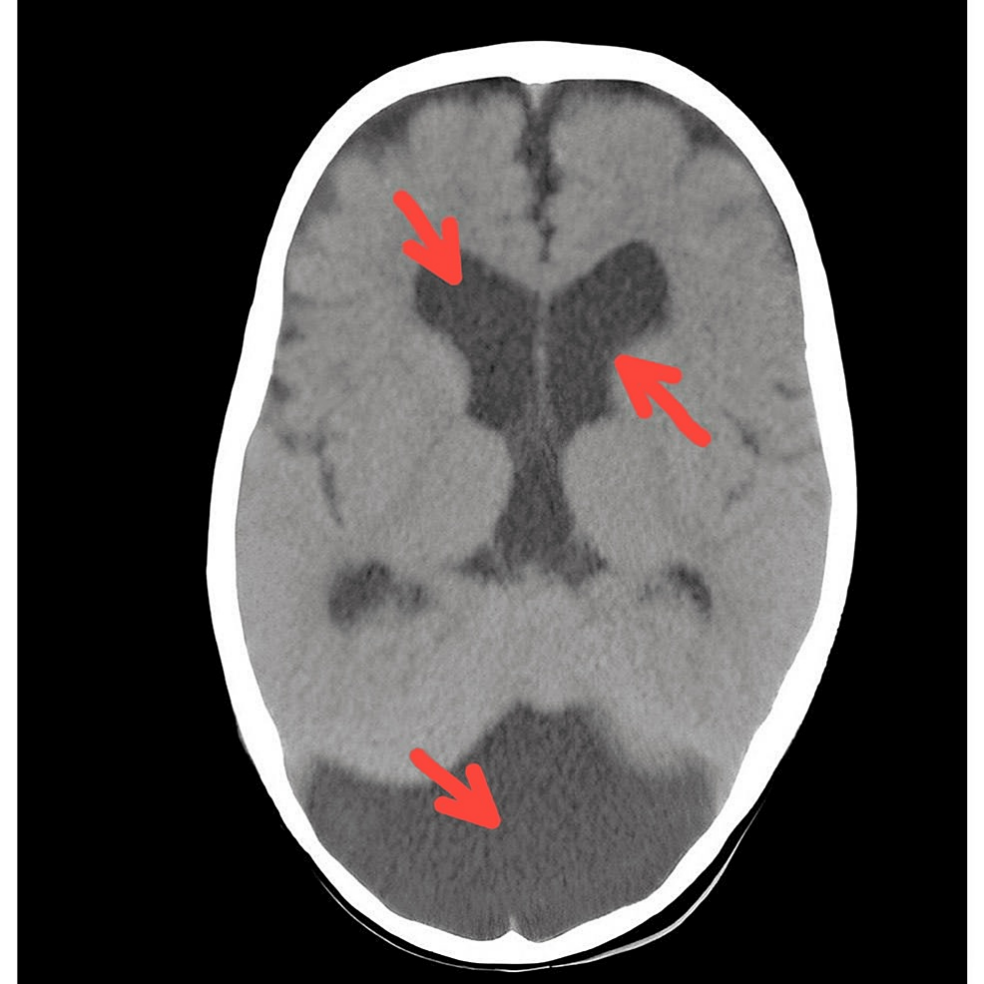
Brain CT scan finding; Enlarged posterior fossa with large cystic space continuous with the fourth ventricle associated with vermis and cerebellar hypoplasia.
